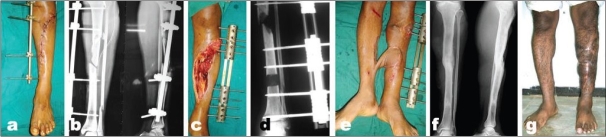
(a) Clinical photograph shows severe open injury of tibia with Ganga Hospital score more than 10. A score above 10 indicates a high velocity injury and primary reconstruction will not be successful. (b) Radiograph (anteroposterior and lateral) shows temporary stabilization. (c) Clinical photograph after debridement. (d) Radiograph (anteroposterior) shows bone transport procedure. (e) Clinical photograph shows cross leg flap. (f) Radiograph (anteroposterior and lateral) shows union at final follow up. (g) Clinical photograph shows weight bearing extremity with limb length equality
